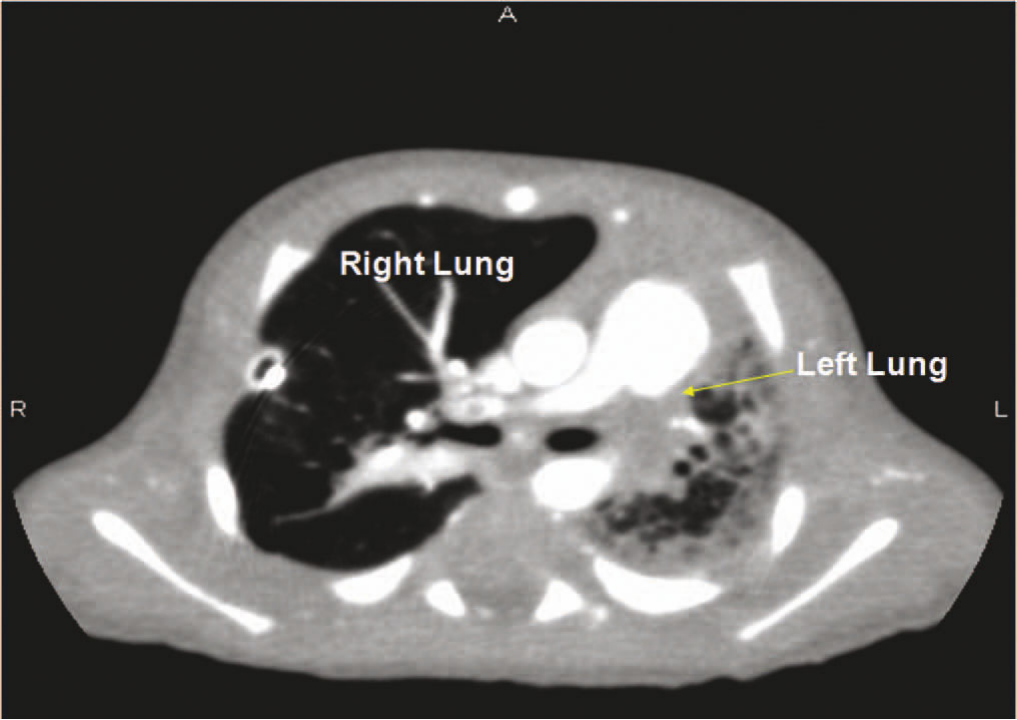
Cross-sectional view on computed tomography angiography confirming hypoplasia of the left lung.
